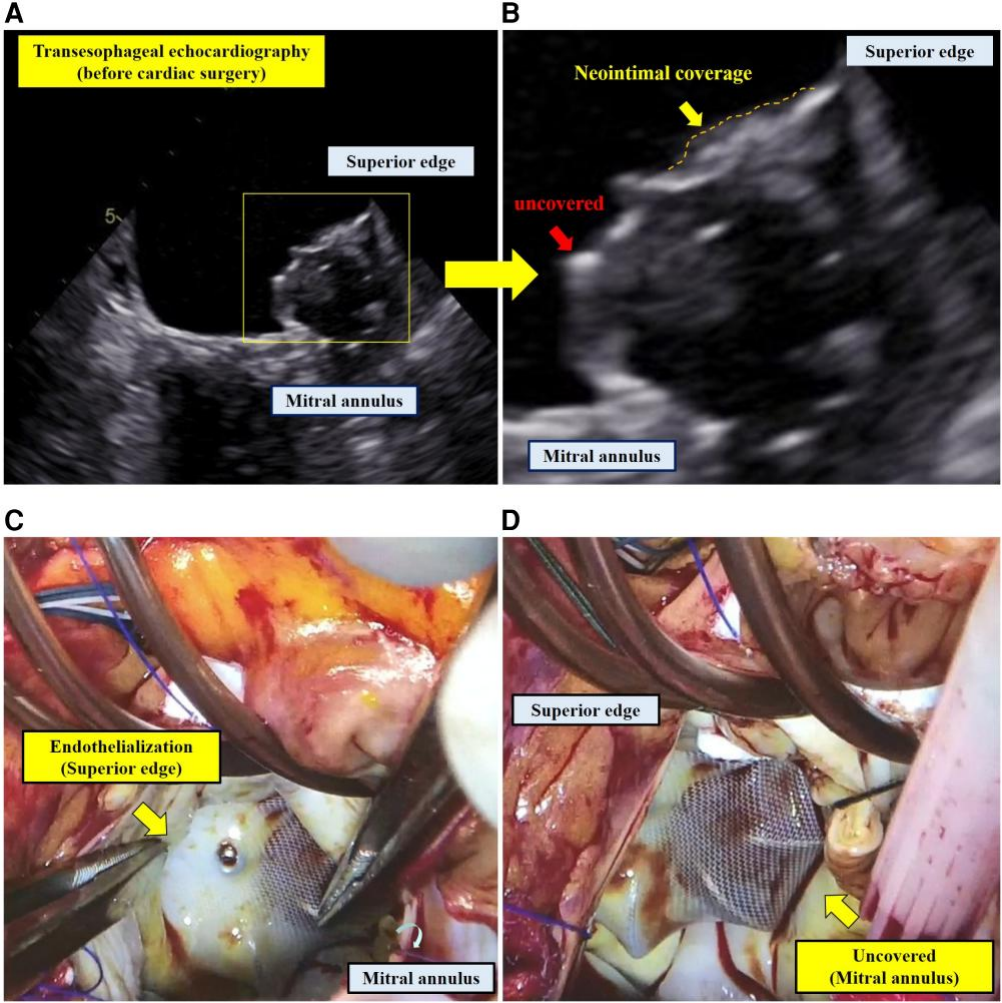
Transoesophageal echocardiography at 2 months before surgery. (*A*) It showed that the intimal coverage was confirmed in the surface in the superior edge of WATCHMAN device, while the device remained uncovered in the mitral annulus side. (*B*) The white and reddish-brown tissue coverage was observed at the superior edge of WATCHMAN device. (*C*) Approximately half of the device remained uncovered in particular at the mitral annulus side.
